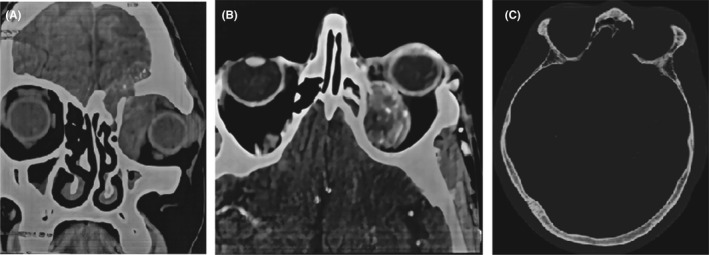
CT orbit coronal cut without contrast (A), axial cut with contrast (B), and axial cut bone window (C) demonstrating the large orbital mass surrounding and indenting the left globe with osteolysis
